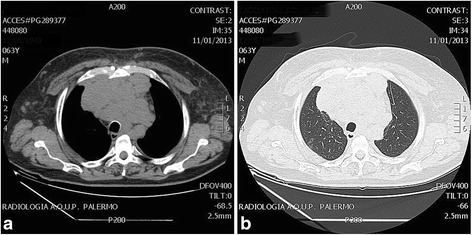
Chest computed tomography scan. a Mediastinal window and b lung window: mediastinal mass 1 month later (axial 12 × 7.5 cm; longitudinal 14 cm). The size of the heteroplasia increased about 10%
